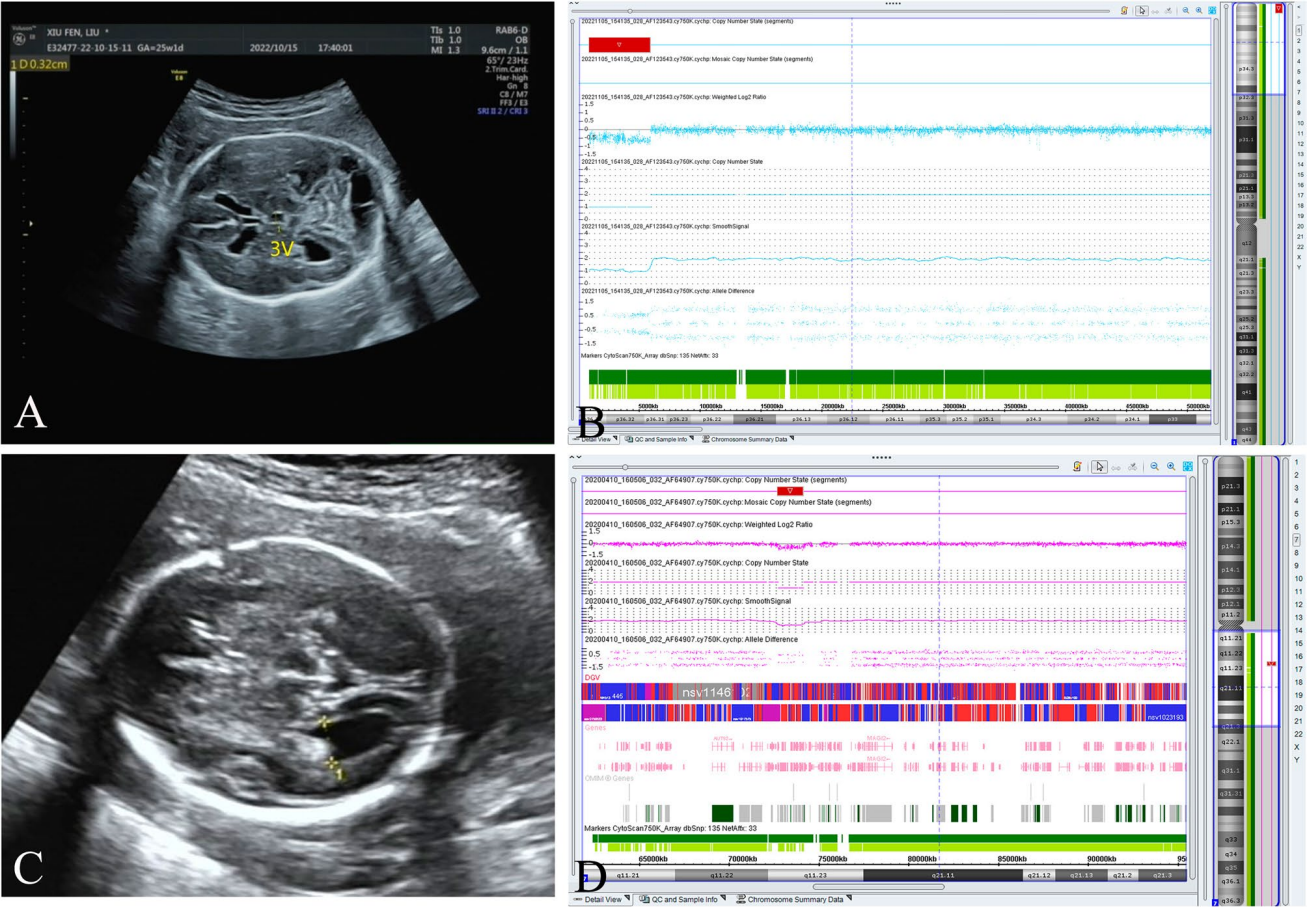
Ultrasound examination and chromosomal microarray analysis results in the enrolled families. (A) Ultrasound examination results showed a ventriculomegaly in Case 3. (B) A 5.0-Mb microdeletion was observed in the fetus of Case 3 using chromosomal microarray analysis (arr[GRCh37] 1p36.33p36.31(849,467_5,866,441) × 1). (C) Dysplasia of corpus callosum was observed in the fetus of Case 6 using ultrasound examination. (D) A 1.4-Mb microdeletion in the 7q11.23 region was detected in the fetus of Case 6 by chromosomal microarray analysis.
